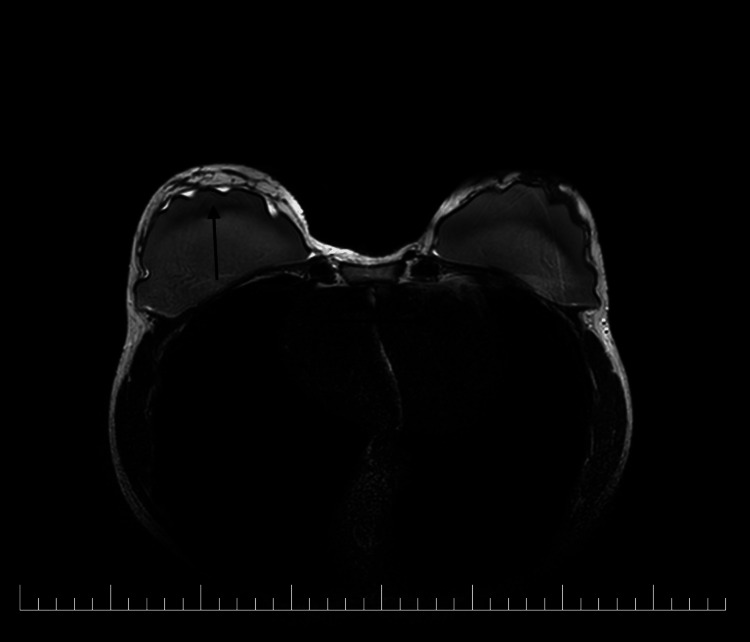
MRI of the breasts 14 months post-augmentation MRI of the breasts showing fluid collection on the right side, with suspicion of intracapsular implant rupture, as indicated by the black arrow. There is a suspected subcapsular rupture of the right implant, particularly in the upper quadrant, with fluid accumulation between the pectoral muscle and the upper edge of the implant.
